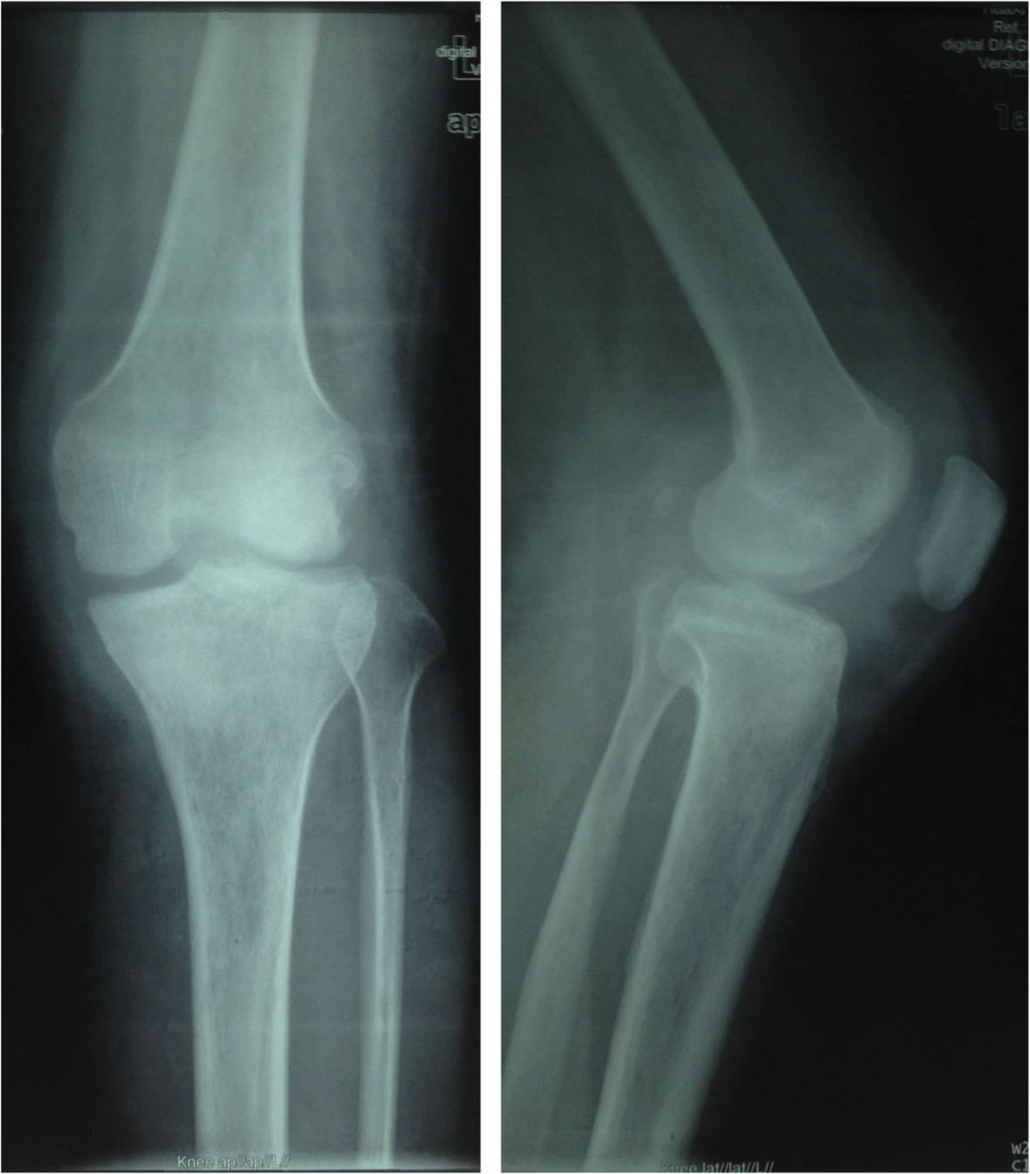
X-ray (anteroposterior and lateral): clearly osteoporosis, uneven joint space narrowing, and joint bone destruction
